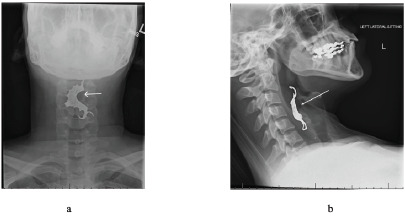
Anterior-posterior (a) and lateral (b) radiographic views of a foreign body (white arrows) in the hypopharynx.
